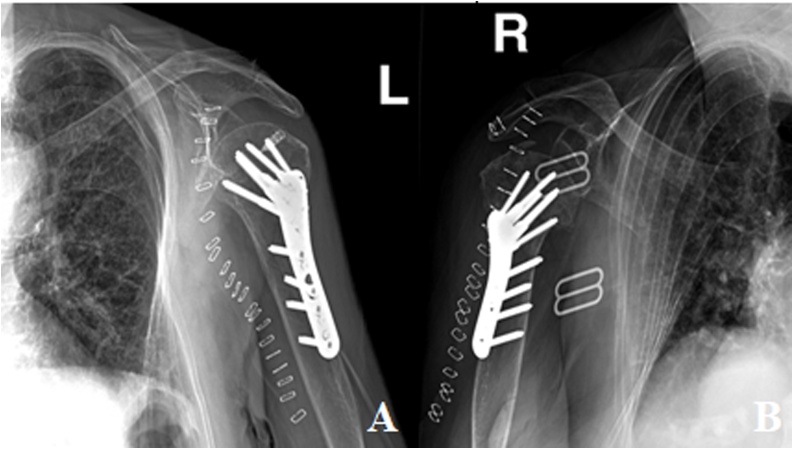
(A) Post-operative X-ray radiograph of the left shoulder showing good alignement post-ORIF of the proximal humerus fracture using plate and ten screws (B) Post-operative X-ray radiograph of the right shoulder showing good alignement post-ORIF of the proximal humerus fracture using plate and ten screws.
